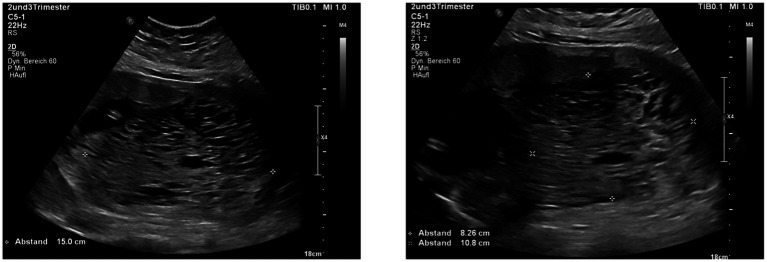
Ultrasound images showing the hydatidiform mole presenting as an enlarged placenta with numerous cysts reminding of a bunch of grapes.
